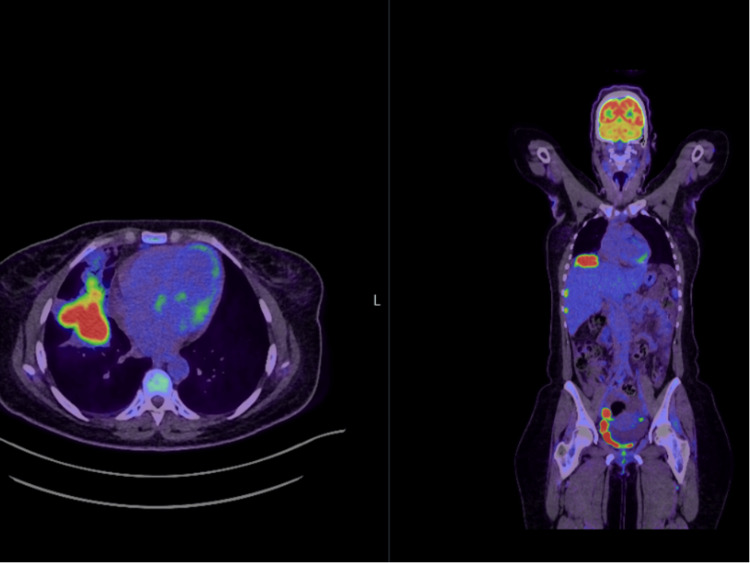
FDG-PET/CT Showing Subphrenic and Pelvic FDG-Avid Lesions Fused FDG-PET/CT image showing intense tracer-avid right pulmonary mass (left: axial view) but on closer inspection located in the subphrenic region (right: coronal view). Additional peritoneal and pelvic foci of uptake are seen, initially suspicious for disseminated malignancy. FDG-PET: fluorodeoxyglucose-positron emission tomography.
